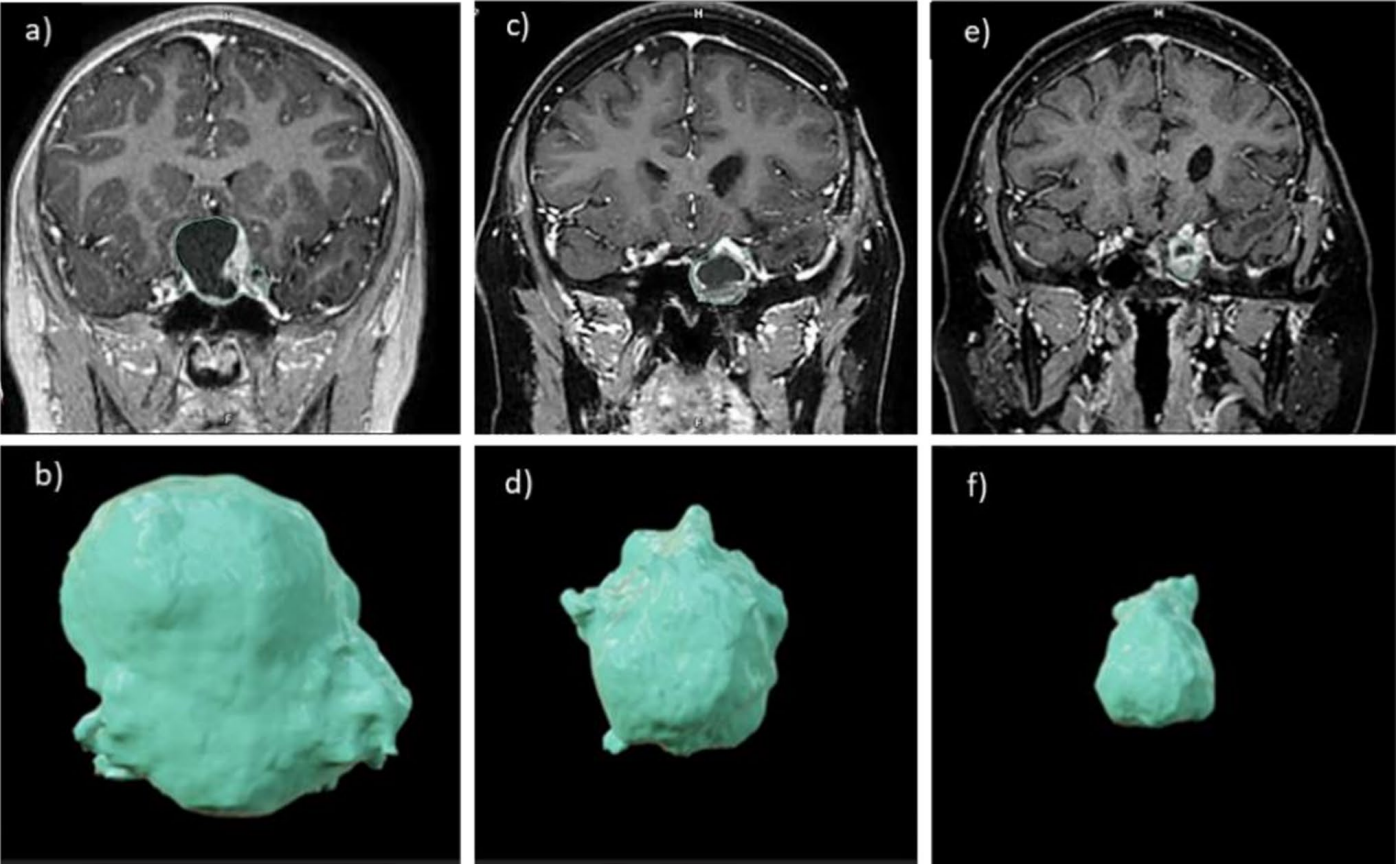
Brain MRI at diagnosis of ACP; b lesion volume at diagnosis, approximately 15 cm^3^; c MRI at the beginning of chemotherapy; d lesion volume of approximately 7 cm^3^ at chemotherapy initiation; e MRI following 12 cycles of temozolomide; and f lesion volume of approximately 3.5 cm^3^ after 12 cycles. ACP, adamantinomatous craniopharyngioma
